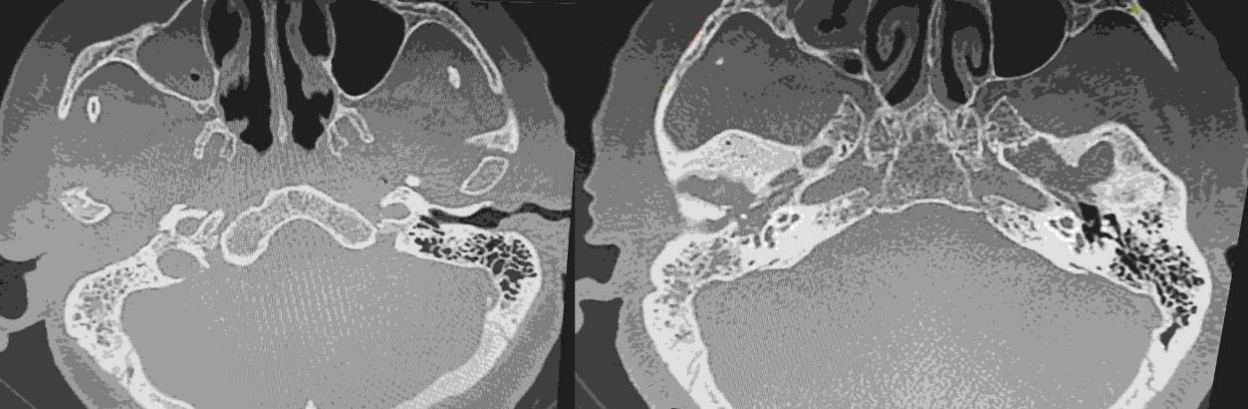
Computed tomography of temporal boned shows soft tissue density in the whole middle ear cavity with expansion in the mastoid and the external auditory canal (EAC), soft tissue ossification elements and smooth erosions of petrous part of temporal bone
